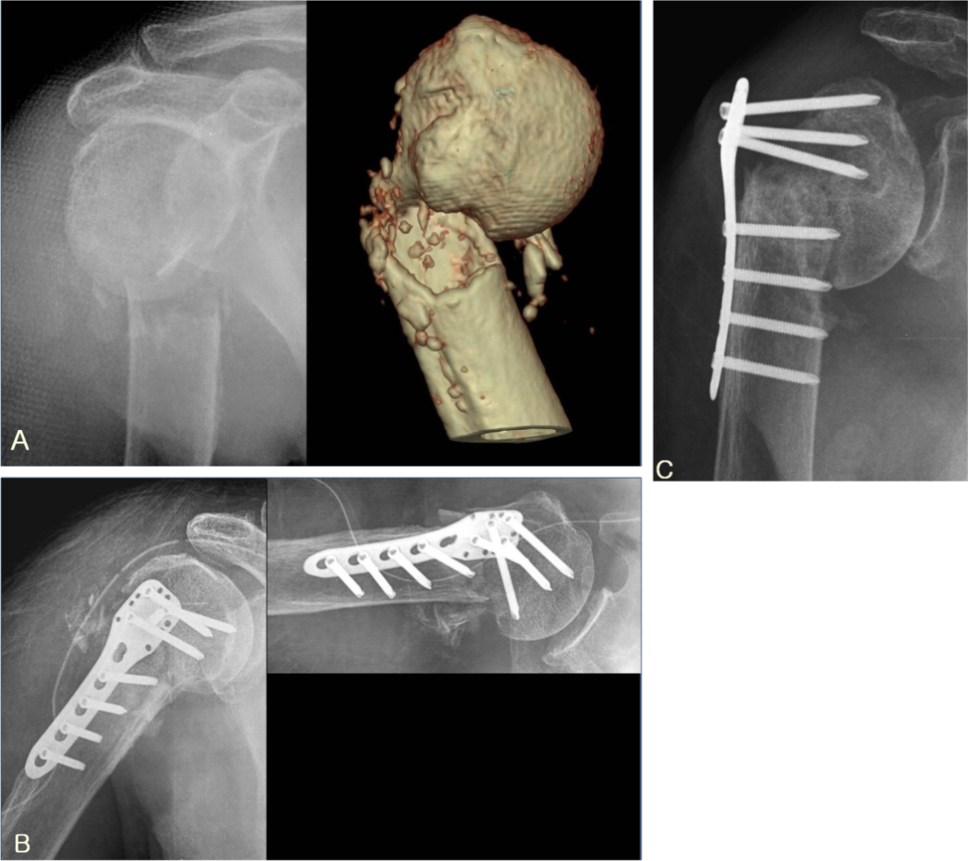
(A) Initial radiographs of 82 years old male with osteoporosis showed 2 part proximal humerus fracture. (B) Immediate postoperative radiographs showed fracture fixation in good alignment but medial support was not achieved. (C) At 4 months after operation, the reduction loss without screw perforation was developed. So, the clinical result was failure.
